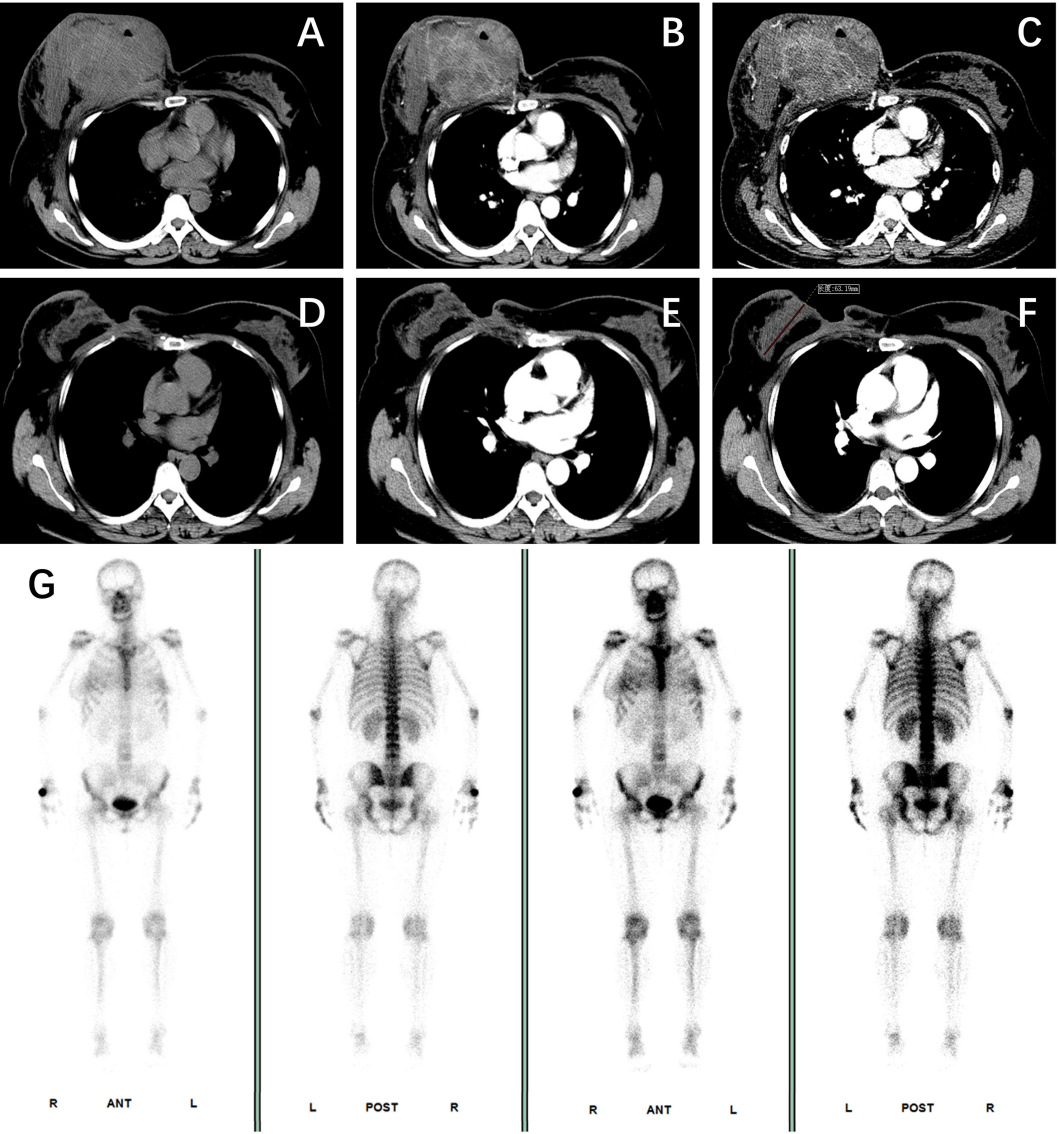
Radiographic response to neoadjuvant therapy. *Baseline (pre-treatment)*: (A-C) Contrast-enhanced axial CT images showing a large, heterogeneously enhancing mass in the right breast, with a maximum diameter of 14.3 cm and visible gas cavities; delayed enhanced CT showed persistent enhancement. *Post-neoadjuvant therapy*: (D-F) Follow-up CECT scans after treatment demonstrated a marked therapeutic response with significant regression of the breast tumor and axillary lymph nodes. (G) Anterior and posterior views from a whole-body bone scan showing no evidence of distant osseous metastases.
